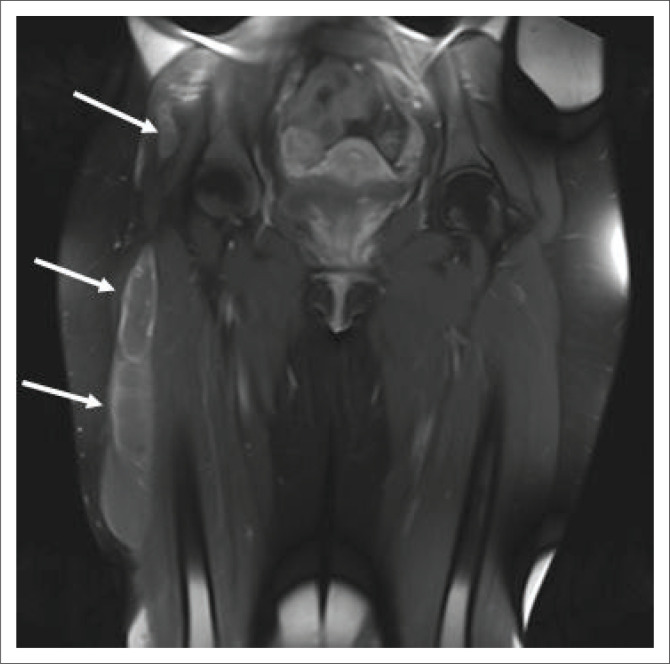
A 20-year-old human immunodeficiency virus-negative female presented with chronic hip pain, which progressively worsened over 3 months. Coronal T1-weighted fat sat post-contrasted magnetic resonance imaging of the pelvis demonstrates rim-enhancing masses within the greater trochanter and intertrochanteric space (images not included), which communicates with the right trochanteric bursa. Rim-enhancing abscesses (solid white arrows) extend from the bursa, inferiorly underneath the ilio-tibial band. Tuberculous osteitis with overlying bursitis and cold abscess formation was confirmed on tissue Gene expert.
